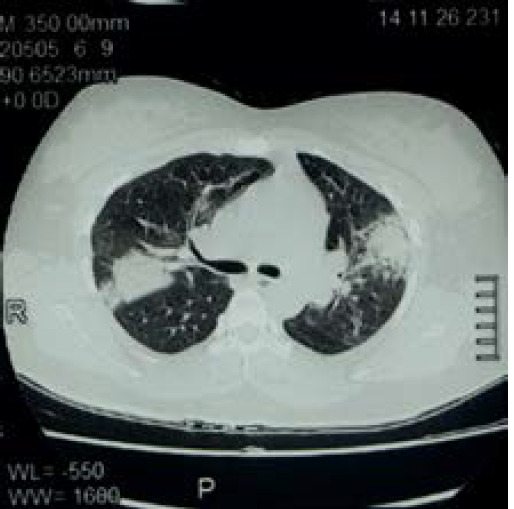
High resolution CT of chest showing bilateral peripheral and peribronchial ground glass opacities with consolidation.
